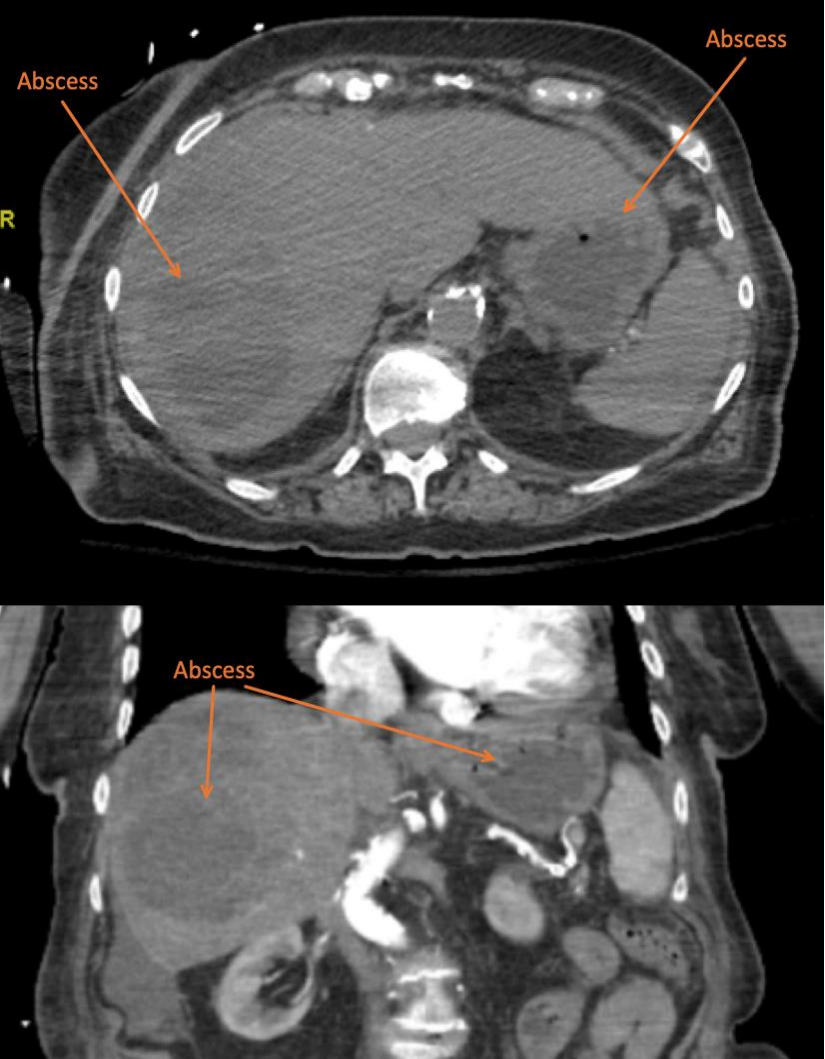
Computed tomography imaging with transverse (top) and coronal (bottom) plane views of the upper abdomen showing large septated fluid collection in the right hepatic lobe, most consistent with a large hepatic abscess, and additional fluid collection in the left hepatic lobe also consistent with hepatic abscess.
